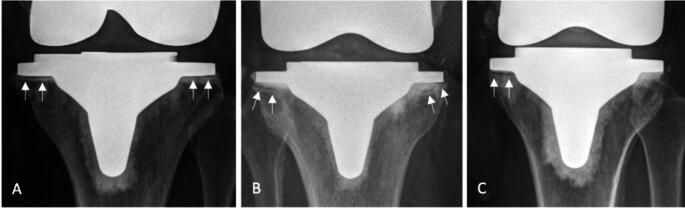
Anteroposterior (AP) radiographs of a 63 years old female patient (A), a 71 years old female patient (B), and a 54 years old female patient (C) of the study cohort following TKA with a cemented fixed bearing cruciate retaining Attune TKA demonstrating the characteristic appearance of tibial radiolucent lines seen in this cohort
